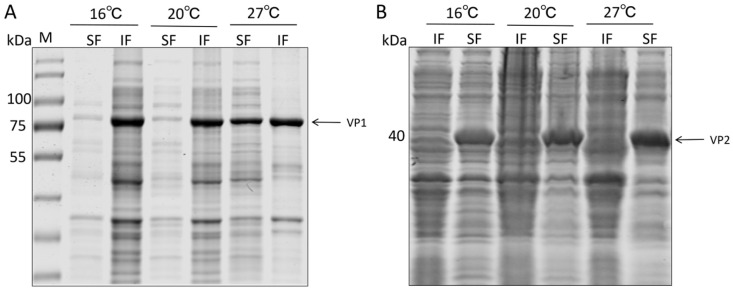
Expression of VP1 and VP2 proteins at different temperatures in the supernatant and inclusion bodies of ultrasonically disrupted *Escherichia coli* cells. SF: Supernatant of ultrasonically disrupted bacterial cells. IF: Inclusion body precipitate after ultrasonic disruption of bacterial cells. (A) Expression of pET32a-VP1 at 16 °C, 20 °C, and 27 °C. (B) Expression of pET24b-VP2 at 16 °C, 20 °C, and 27 °C.
